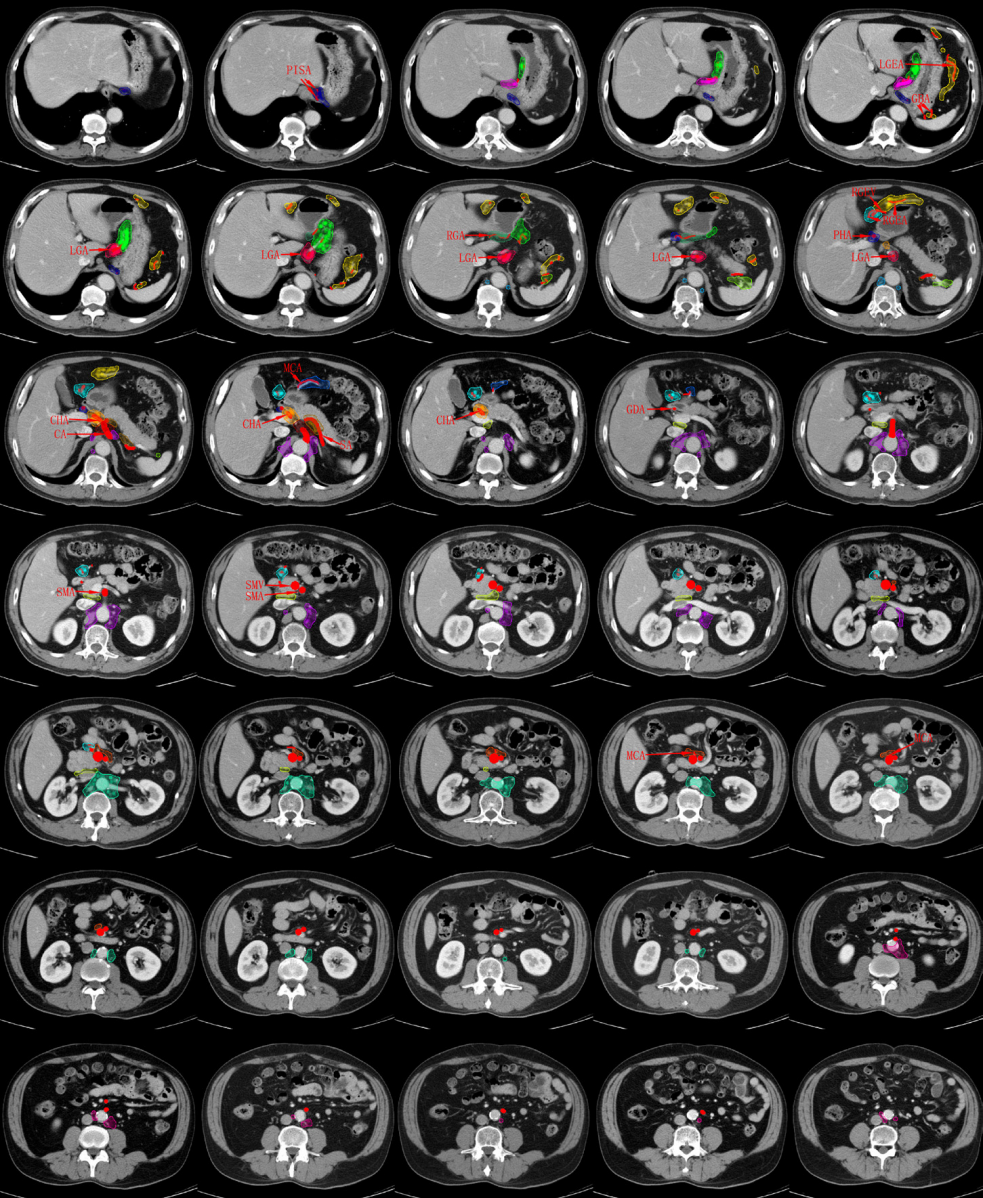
Consistency mapping of 16 lymph node stations in gastric cancer by CT-based vessel-guided delineation of 255 patients In total, 35 representative axial CT images were selected moving in the cranial to caudal direction in 5 mm slices. PISA: phrenica inferior sinistra artery; LGA: left gastric artery; RGA: right gastric artery; GBA: gastricae breves artery; LGEA: left gastroepiploic artery; RGEA: right gastroepiploic artery; RGEV: right gastroepiploic vein; PHA: proper hepatic artery; CHA: common hepatic artery; CA: celiac artery; SA: splenic artery; MCA: middle colic artery; GDA: gastroduodenal artery; SMA: superior mesenteric artery; SMV: superior mesenteric vein.
